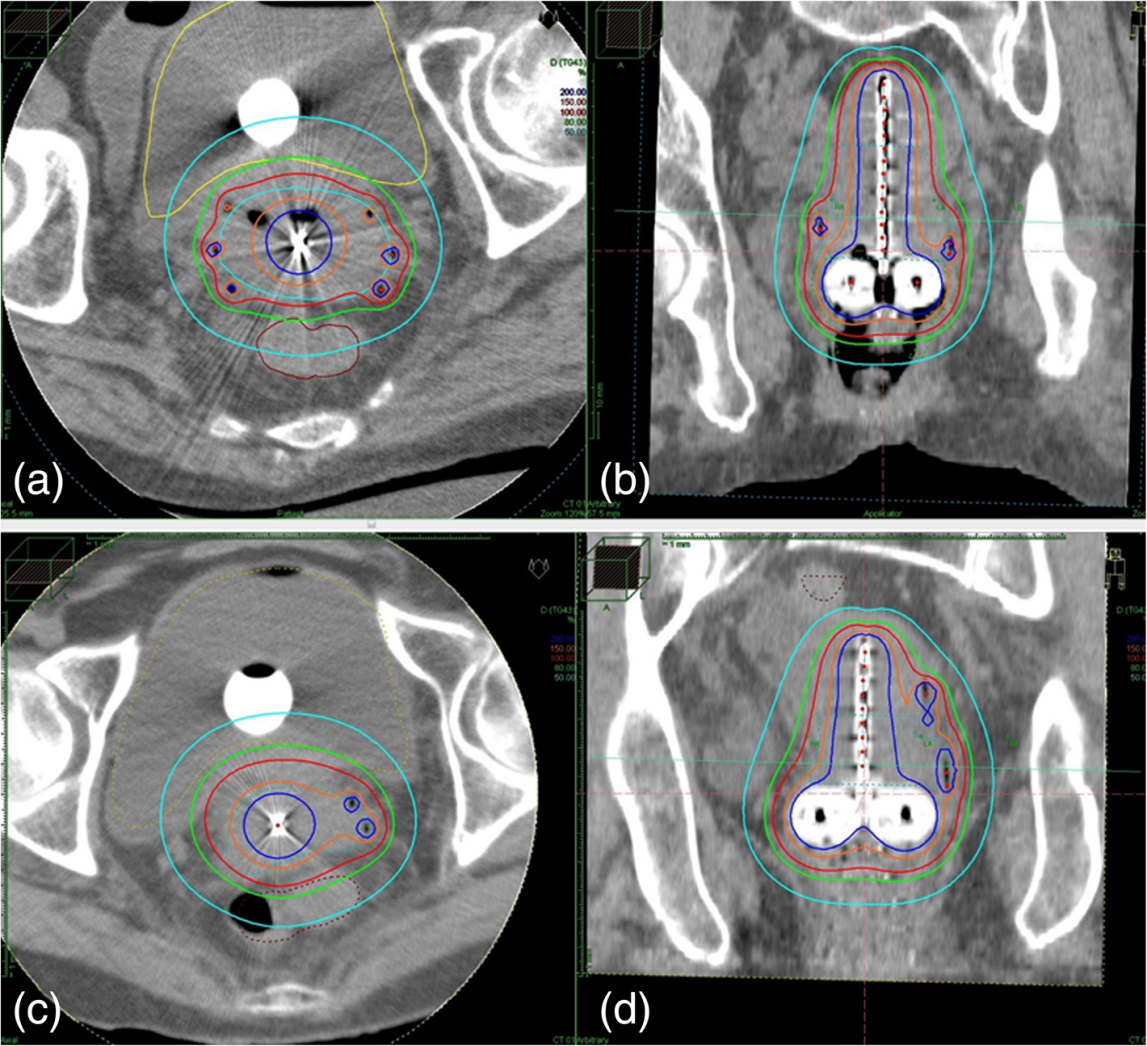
Examples of dose distributions of computed tomography-based hybrid intracavitary and interstitial brachytherapy (HBT). a axial and (b) coronal images of HBT for a IIIB patient with bilateral parametrial invasion. In each side of the parametrium, three additional interstitial needles were inserted to cover lateral tumor extension. c axial and (d) coronal image of HBT for a IIIB patient with left parametrial invasion. Two interstitial needles were inserted in the left parametrium
